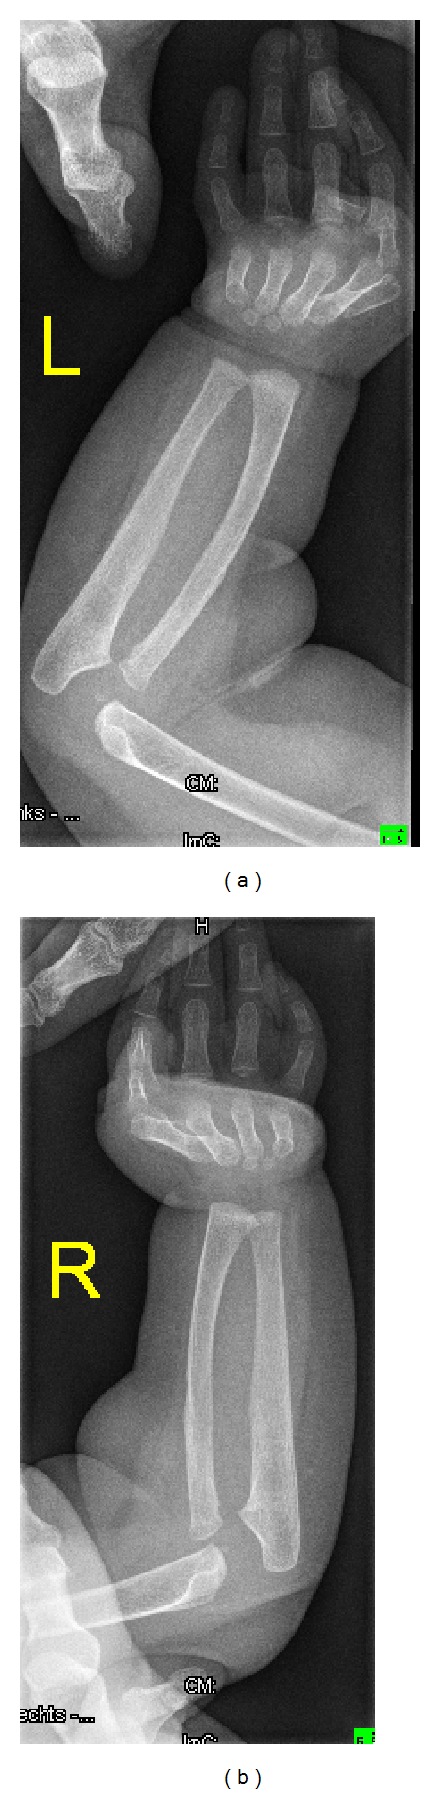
(Patient 2) AP forearms radiograph showed bilateral dislocation of the elbows, dysplastic ulnae, and brachydactyly.
